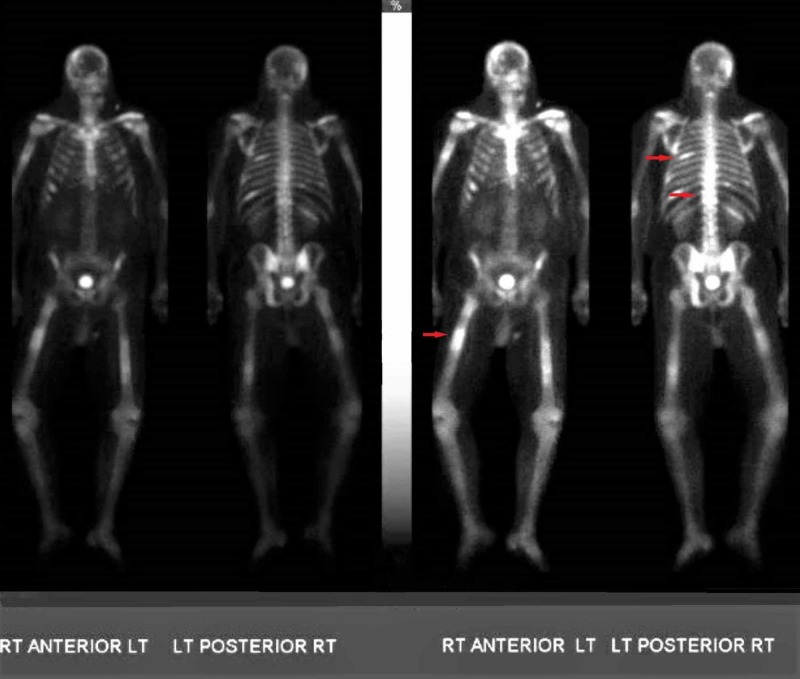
The PET-CT scan showing hypermetabolic activity involving the T12 vertebral body and paravertebral soft tissues, right femoral shaft, and left sixth rib
